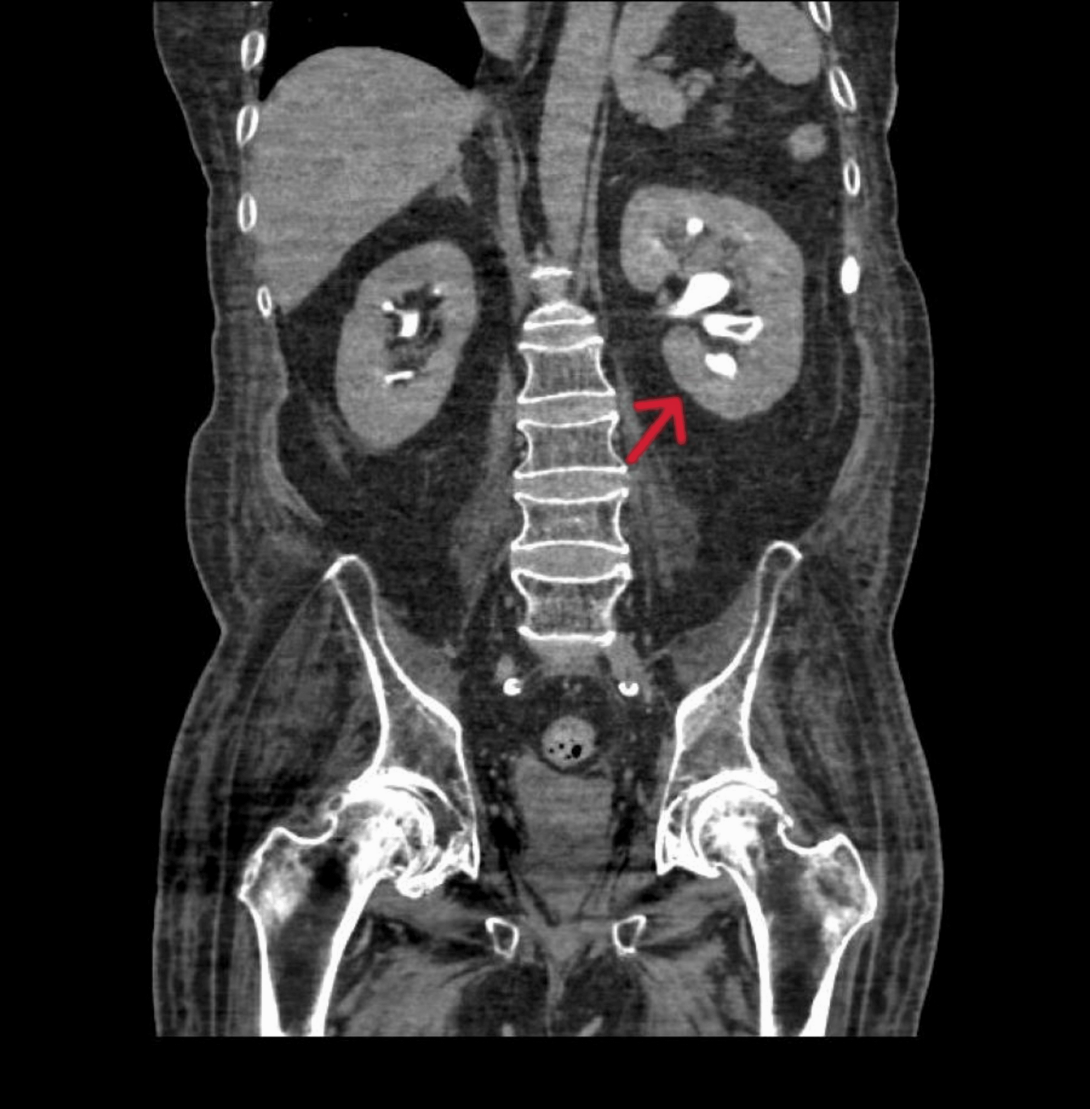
CT urogram demonstrating mild left-sided hydroureteronephrosis with urothelial thickening
